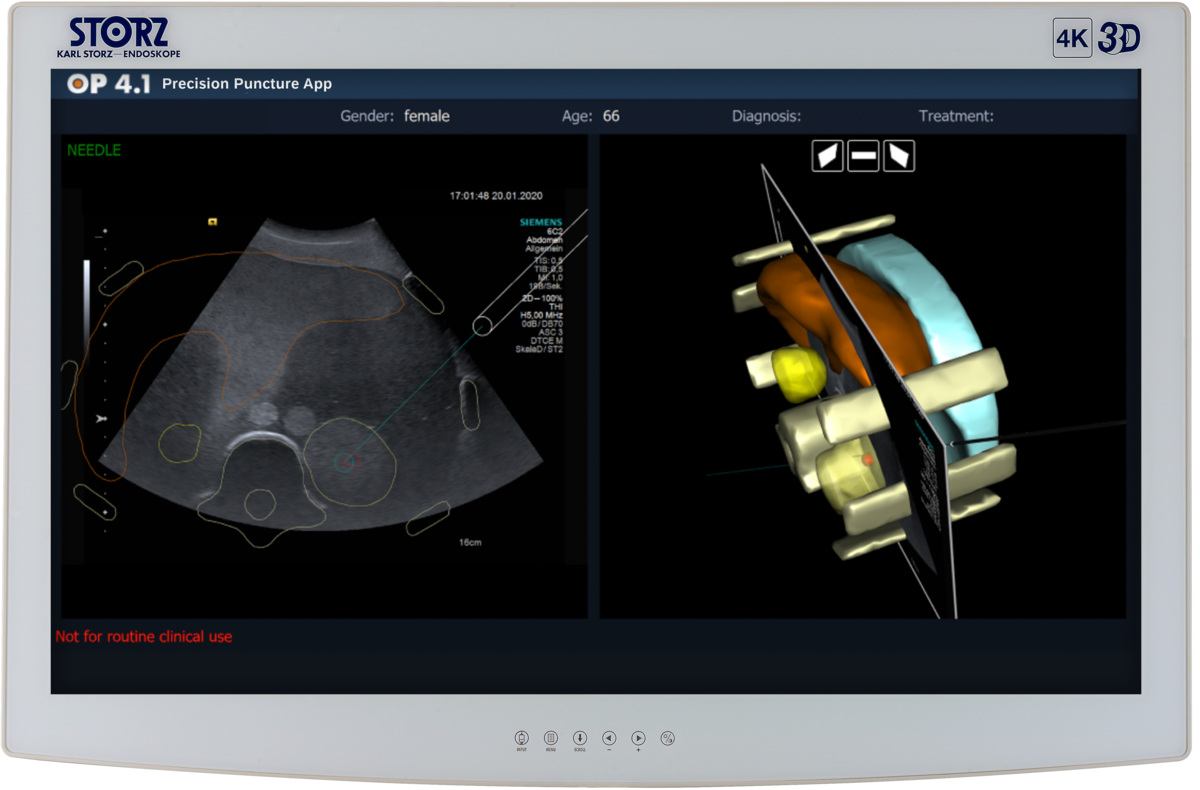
OP 4.1 precision puncture app. By combining live ultrasound images with segmented computed tomography images from connected systems through the central OP 4.1 platform, this app enables a safe and fast percutaneous puncture of target structures, for example, in the kidney. Left: ultrasound image with the projection of the needle and the segmented structures from the computed tomography images. Right: 3D scene, including the ultrasound plane and the segmentations, made possible by multimodal image fusion.
